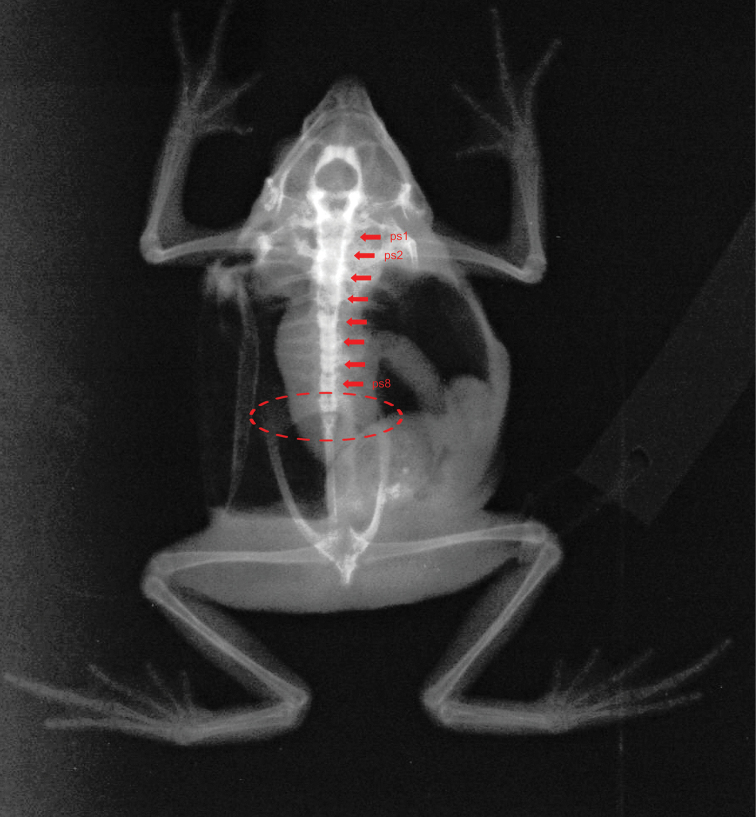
X-ray of the paratye *Rhinella
